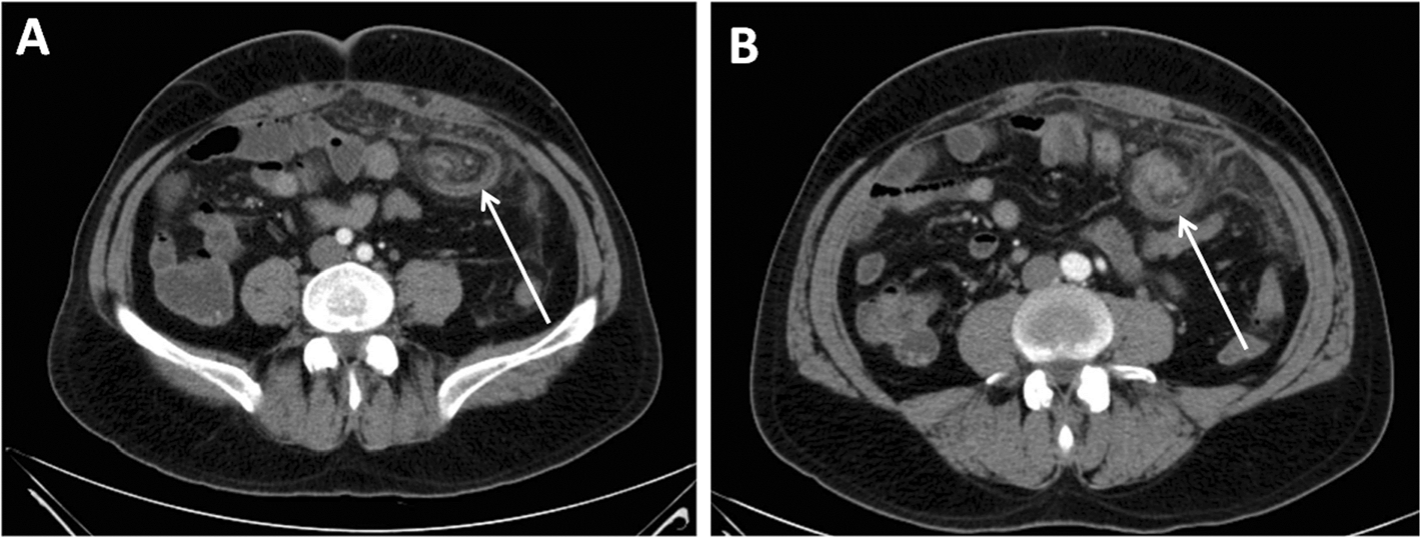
Computed tomography scans showing the whirl (arrow) of the omentum. A) caudal view. B) cranial view.
